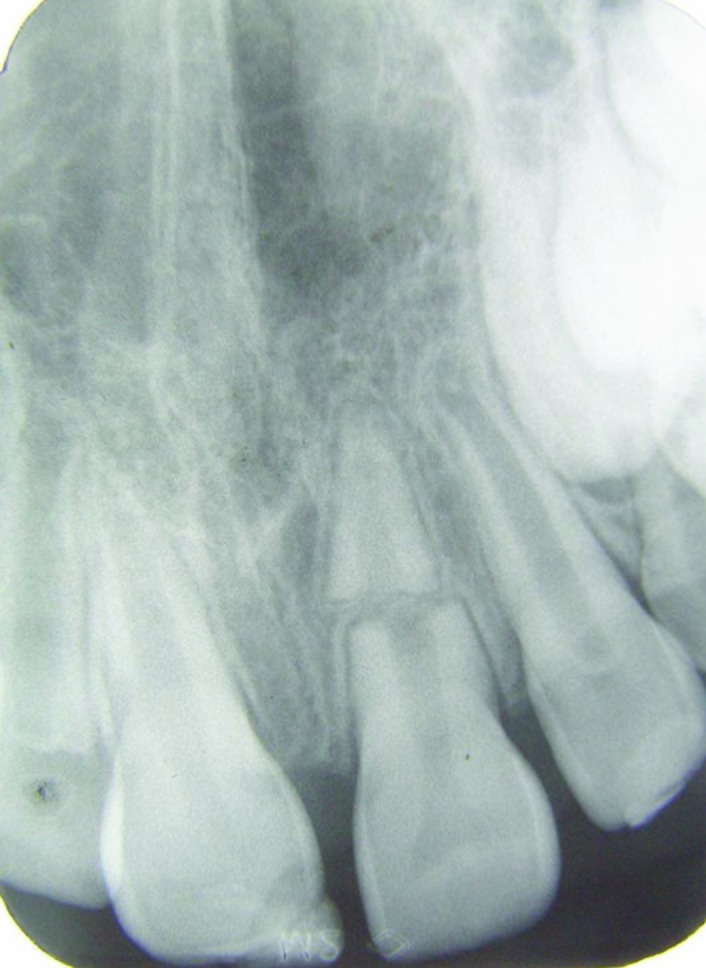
At the 24-month recall, the left central incisor had no mobility and pain in horizontal and vertical percussion tests. The crown was not discolored and the occlusion was normal. The tooth also responded normally to the electrical and thermal stimulation tests. In the radiographic examination, not only the thickening of dentinal walls of the root, but also the closure of apex, was noticeable. Healing of the fracture with calcified tissue was evident. External surface resorption (peripheral rounding), mesial and distal to the fracture site, which is a characteristic finding in horizontal root fracture, was detected radiographically (Figure 6).
